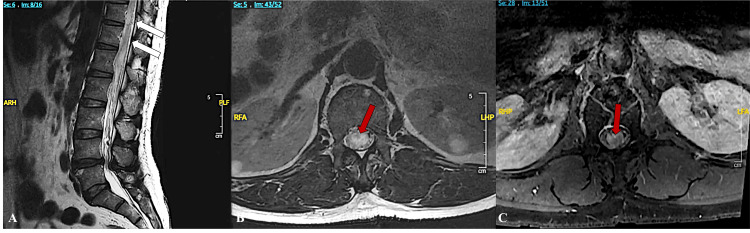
Preoperative magnetic resonance imaging (MRI). Preoperative sagittal T2 sequence MRI (A) exhibited thoracolumbar spinal cord signal change consistent with spinal cord edema. T2 sagittal MRI imaging demonstrates intradural vessels overlying the spinal cord (white arrows). Axial T2 (B) and T1 (C) sequence views at the L1 vertebral level also show central cord signal change (red arrows). Images (A) and (B) have been lightened for clarity.
